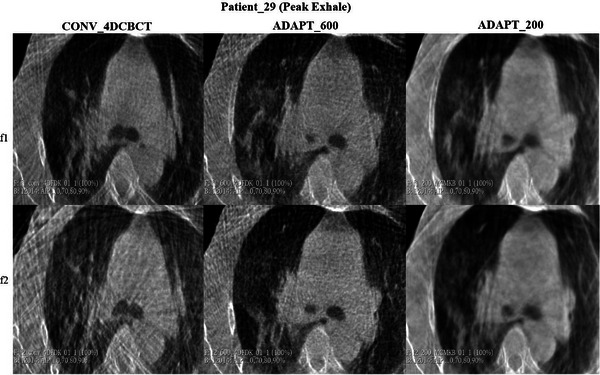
Visual assessment of tumor motion for patient_29 during peak exhale phase. The comparison includes conventional 4DCBCT, ADAPT_600, and ADAPT_200 scans across fractions 1 (f1) and 2 (f2).
